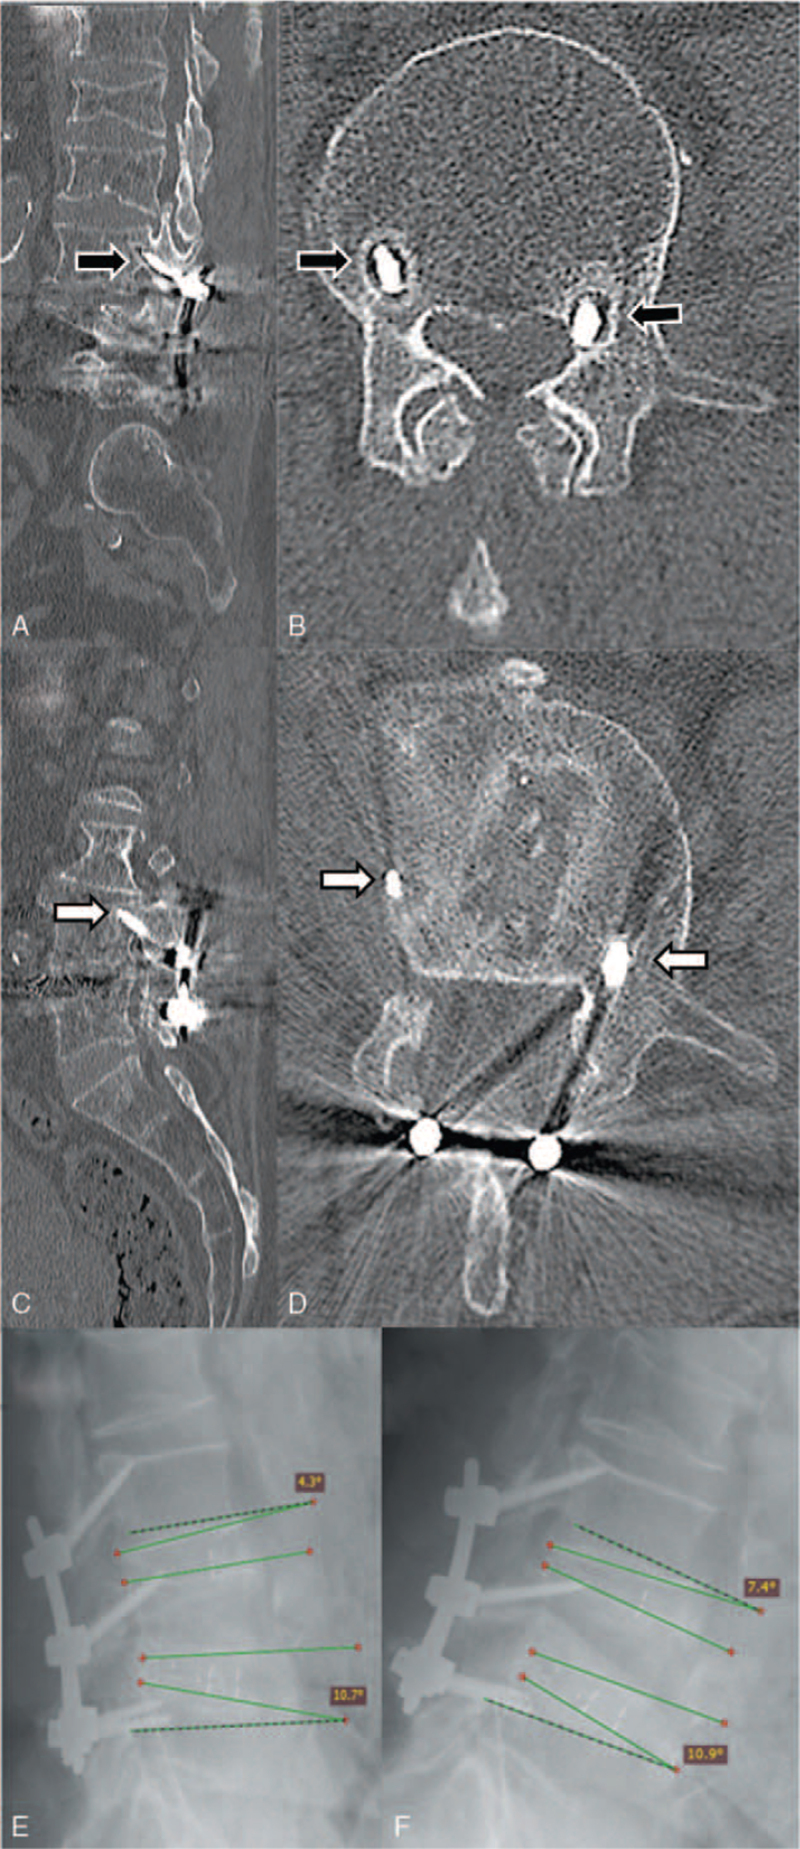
Images of a patient after L3–L4–L5 surgery show representative complications. (A, B) Sagittal and axial computed tomography scans show evidence of haloing around the screws through the L3 pedicles (black arrows). (C, D) Sagittal and axial computed tomography scans show no loosening of the screws through the L4 pedicles (white arrows). (E, F) Sagittal dynamic X-rays in (E) extension and (F) flexion show screw mobility at the L3 to L4 level.
